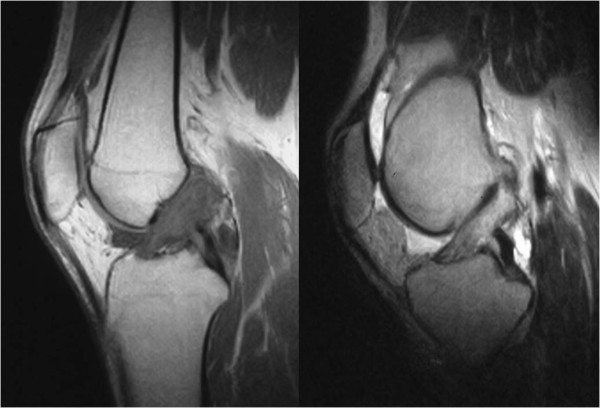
Preoperative MRI image. ACL presented signal changes of iso- and high signal intensity at T1WI (left) and at high signal intensity at T2WI (right), and also demonstrated swelling.
